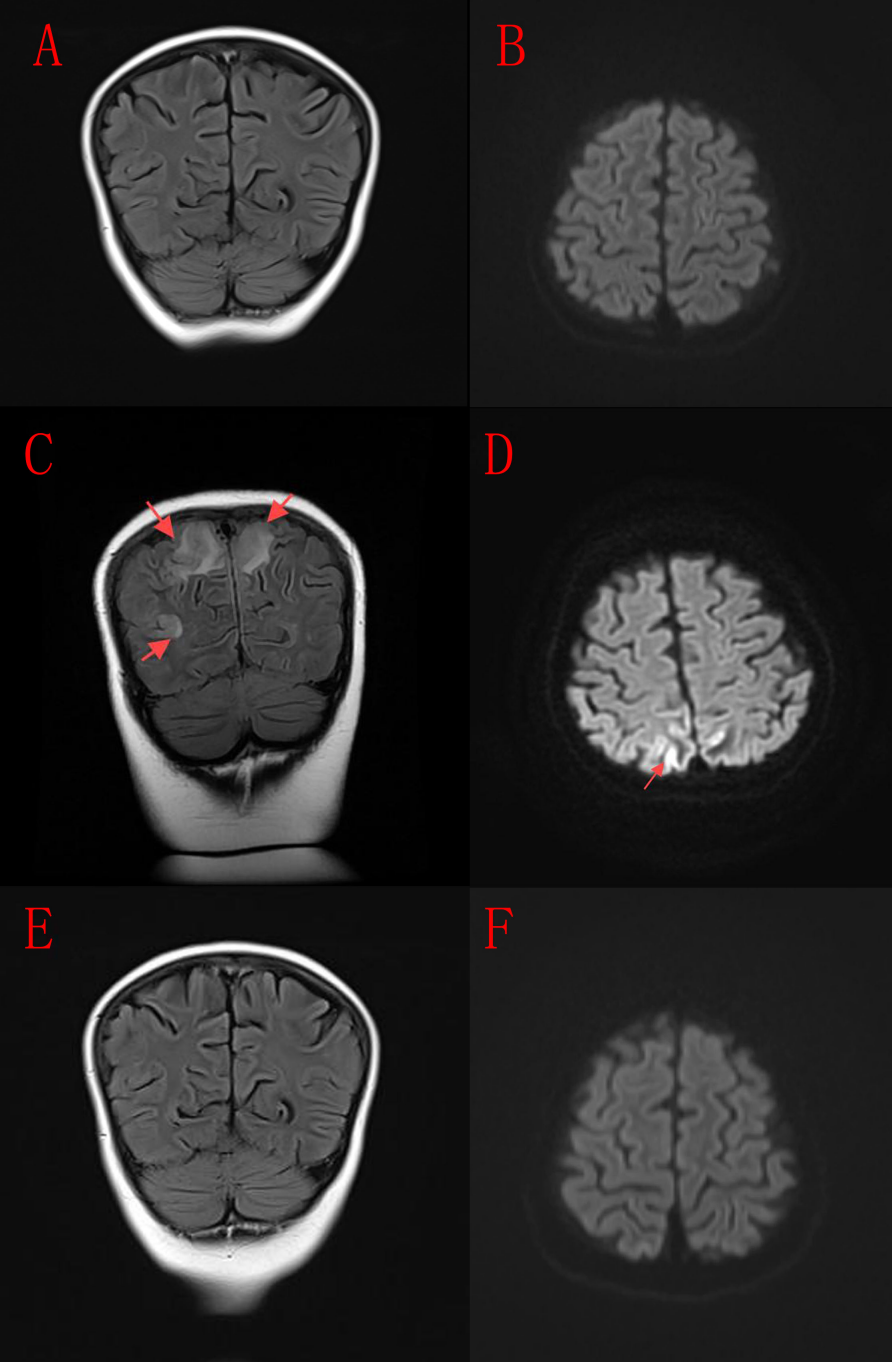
Neuroimaging findings of the patient. (A, B) Initial cranial MRI scan obtained prior to the onset of neurological symptoms. (C, D) Follow-up cranial MRI after the patient developed neurological symptoms, including convulsions, impaired consciousness, and coma, revealing hyperintense signals on FLAIR and DWI sequences in the bilateral occipital and parietal lobes. (E, F) Cranial MRI following antihypertensive therapy, demonstrating near-complete resolution of the abnormal signals.
